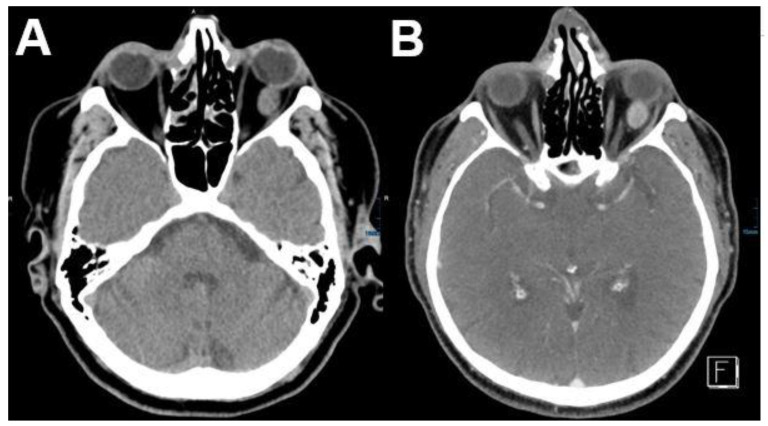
Cavernous hemangioma.* CT of a 54-year-old female patient with a left-sided cavernous hemangioma. The tumor appears as an intraconal, well-circumscribed, slightly hyperdense mass (A) with mild contrast enhancement (B).
